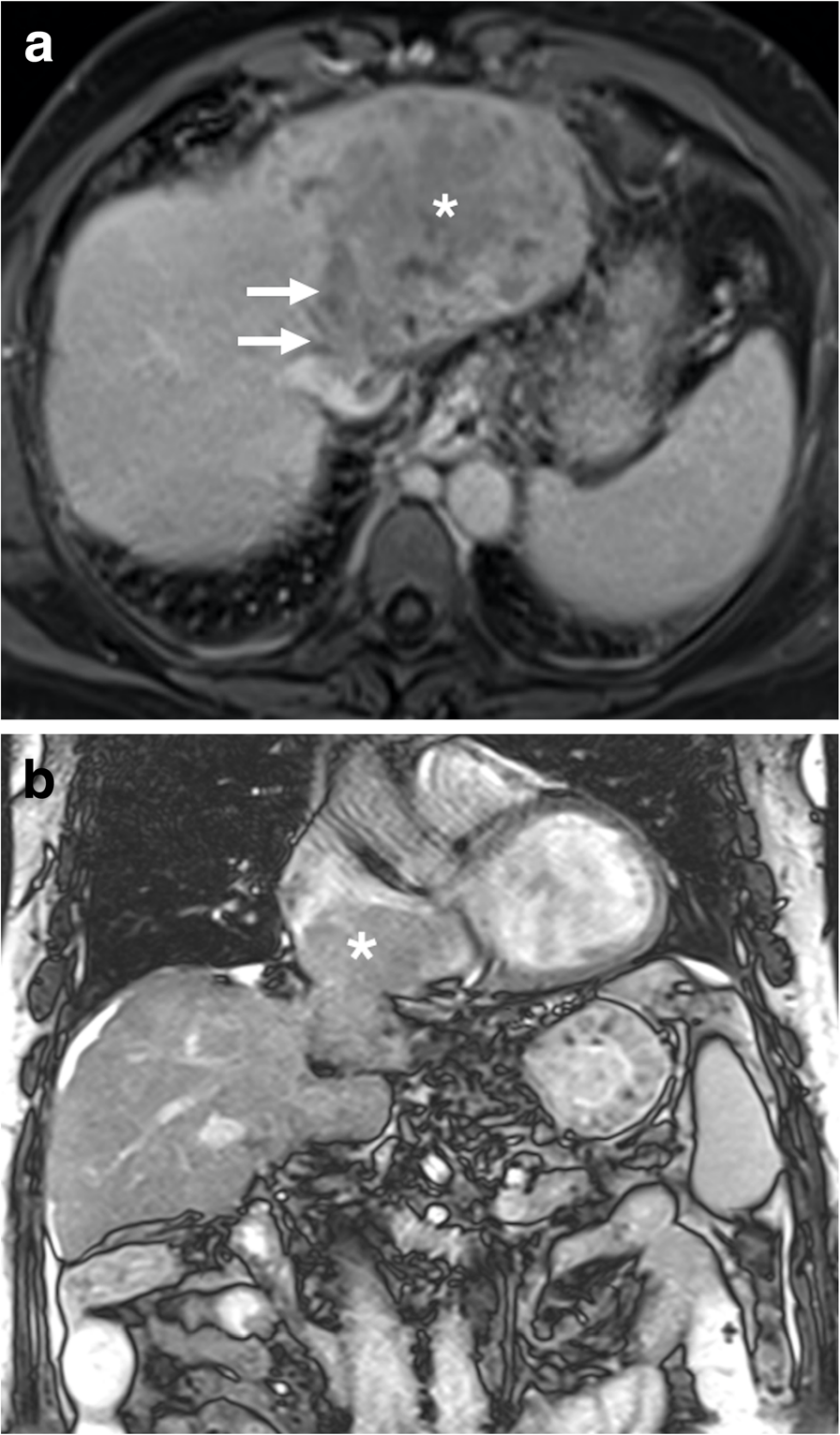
A 60-year-old male with known hepatitis B-related chronic parenchymal liver disease now presents with abdominal pain, low extremity edema, elevated liver enzymes, and elevated AFP levels. a Axial plane postcontrast T1W MR image demonstrates a heterogeneously enhancing solid mass within the left liver lobe (asterisk) with extending tumor thrombus to the left hepatic vein (arrows) and IVC. b Coronal T2W MR image of the same patient demonstrates the extension of the tumor thrombus in the right atrium (asterisk)
